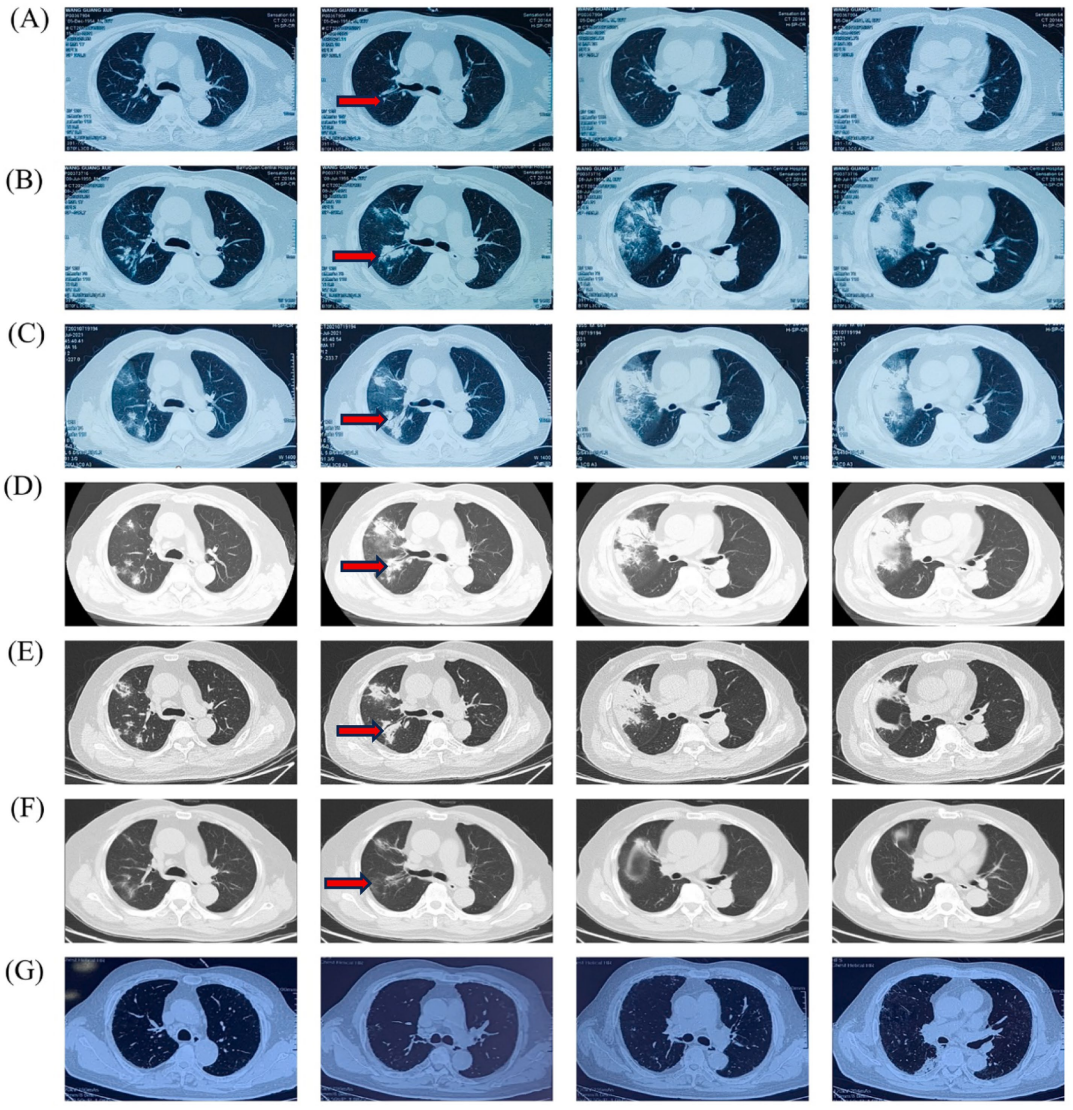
Timeline of the patient’s Chest HRCT imaging scan: (A) 15 June 2021, (B) 8 July 2021, (C) 19 July 2021, (D) 26 July 2021, (E) 2 August 2021, (F) 11 September 2021, (G) 4 July 2022.
